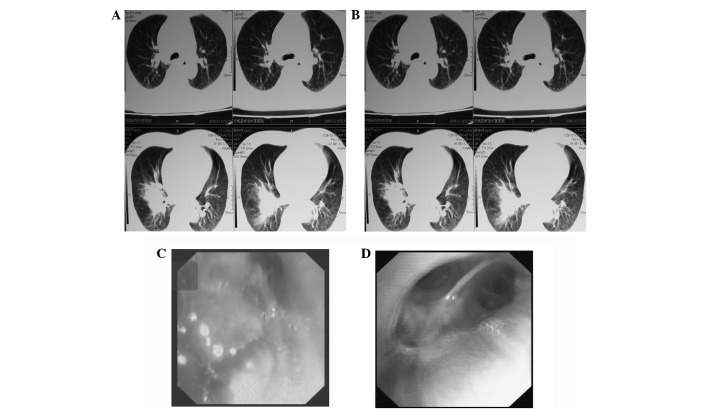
Case 2. (A) Computed tomography (CT) imaging prior to treatment (3/2/2009); (B) CT imaging following treatment (10/20/2010); (C) upper lobe of the right lung prior to treatment (3/11/2009); (D) upper lobe of the right lung following treatment (10/20/2010).
